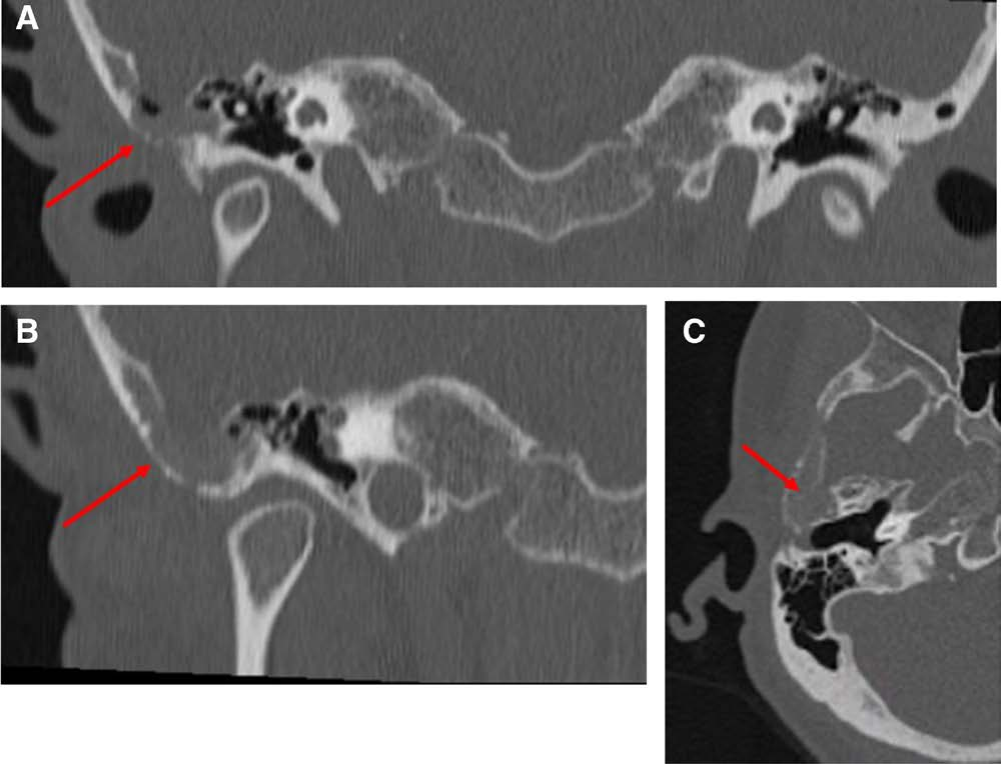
CT scan without contrast. A, B, Coronal images, red arrow indicates the area of the lytic bone lesion within the temporal bone. C, Axial image, red arrow indicates the area of a lytic bone lesion.
